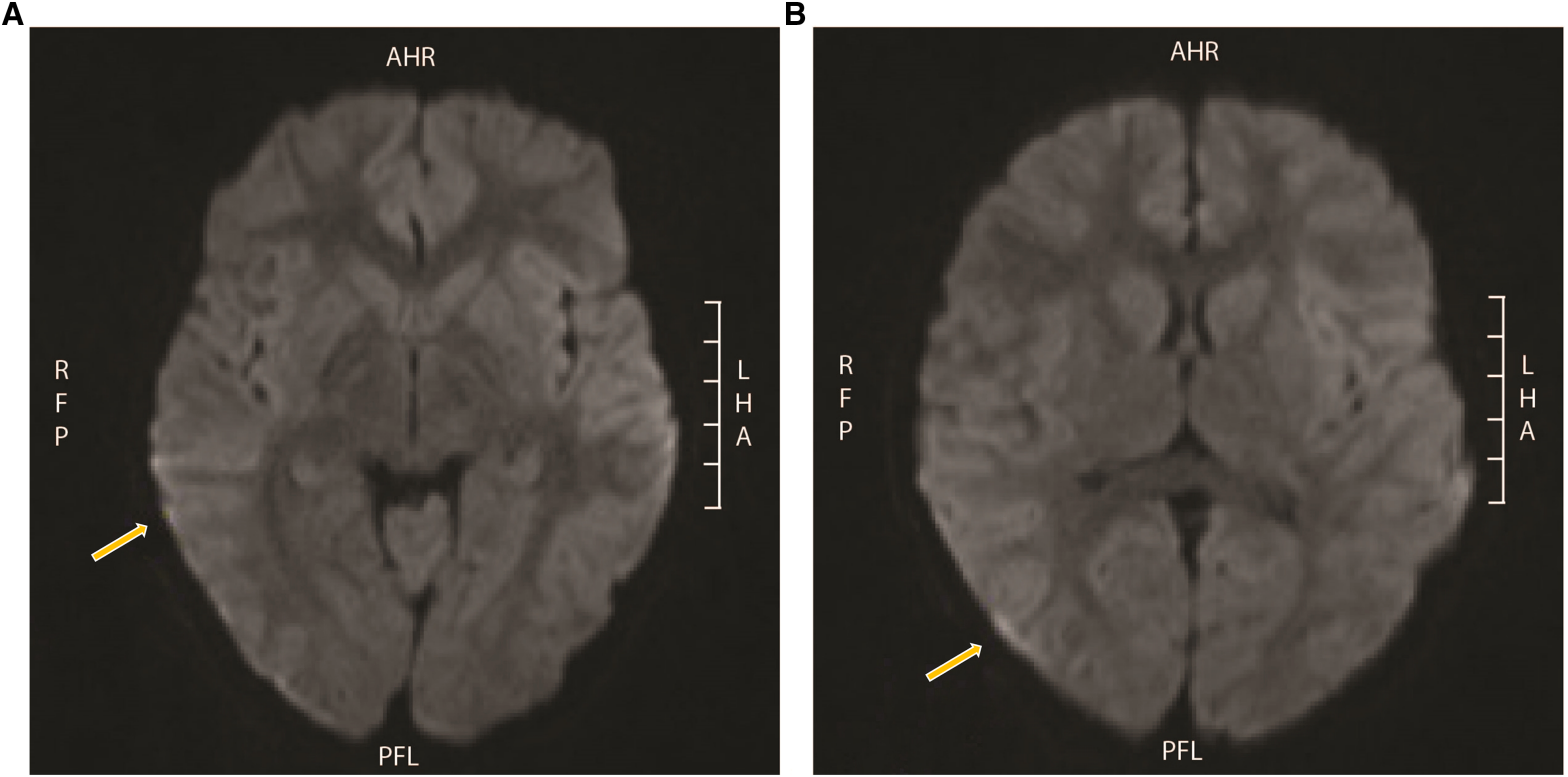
MRI from patient 1. Hypersignal on FLAIR within the sulci of the bilateral cerebral hemispheres, especially in the (A) posterior parietal and (B) occipital regions with minimal restricted diffusion in the extra-axial spaces of the bilateral temporal and occipital regions, and also increased linear enhancement in the sulci that is likely vascular and suggests the presence of hyperemia. MRI, magnetic resonance imaging; FLAIR, fluid-attenuated inversion recovery.
